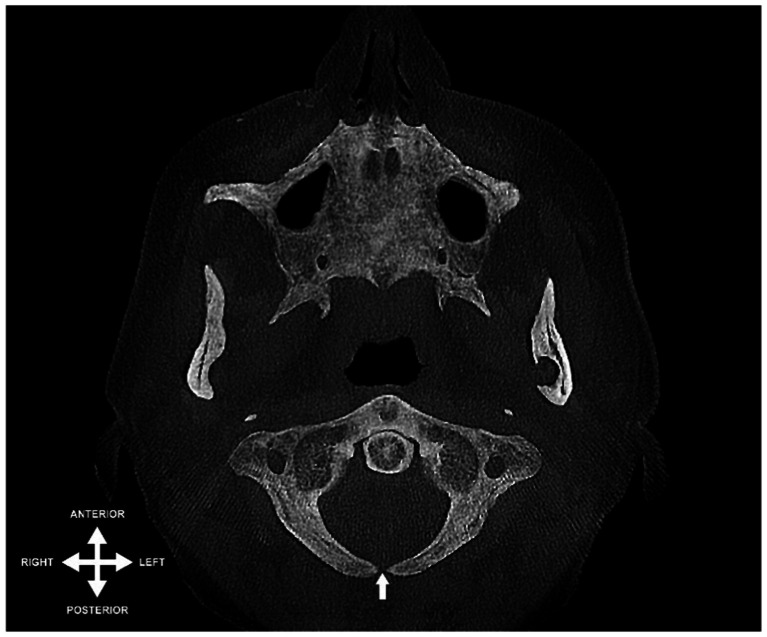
Axial view of a CBCT scan depicting midline cleft in the posterior arch of the atlas (indicated by arrow) – an instance of Currarino’s type A variation.
